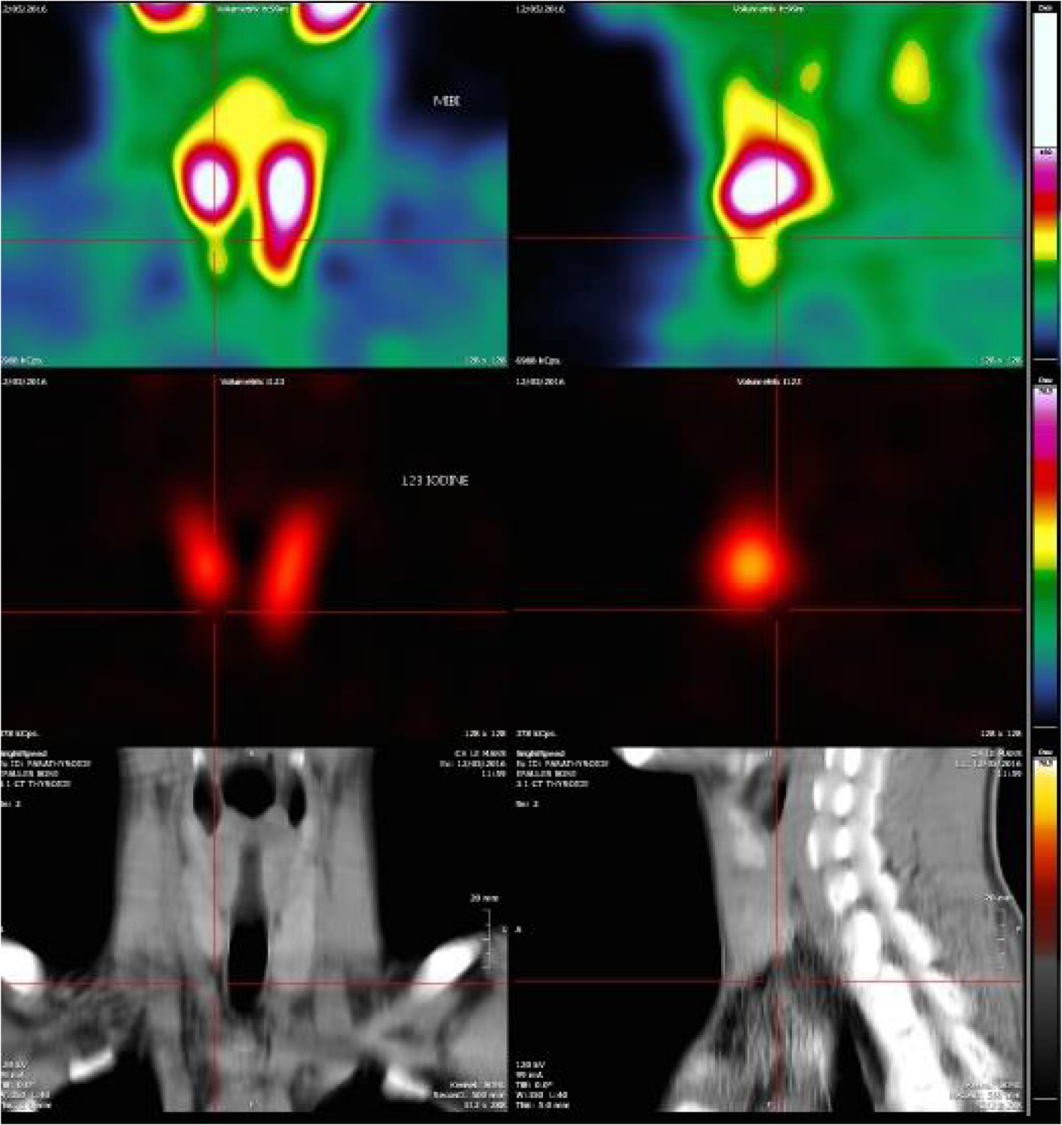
Parathyroid scintigraphy: substraction images are compatible with the presence of bilateral inferior parathyroid adenomas
